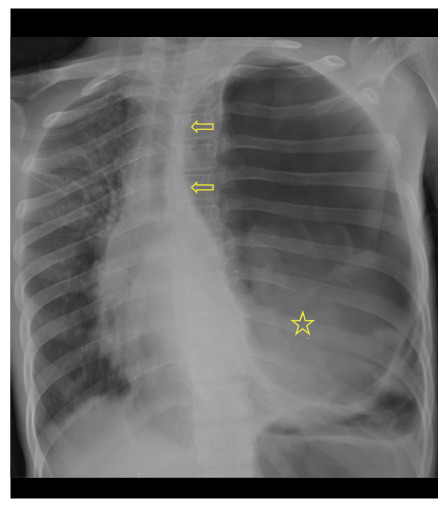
Chest radiograph showing a tension pneumothorax on the left, mediastinal shift to the right (arrows), and a ruptured hydatid cyst (asterisk).
